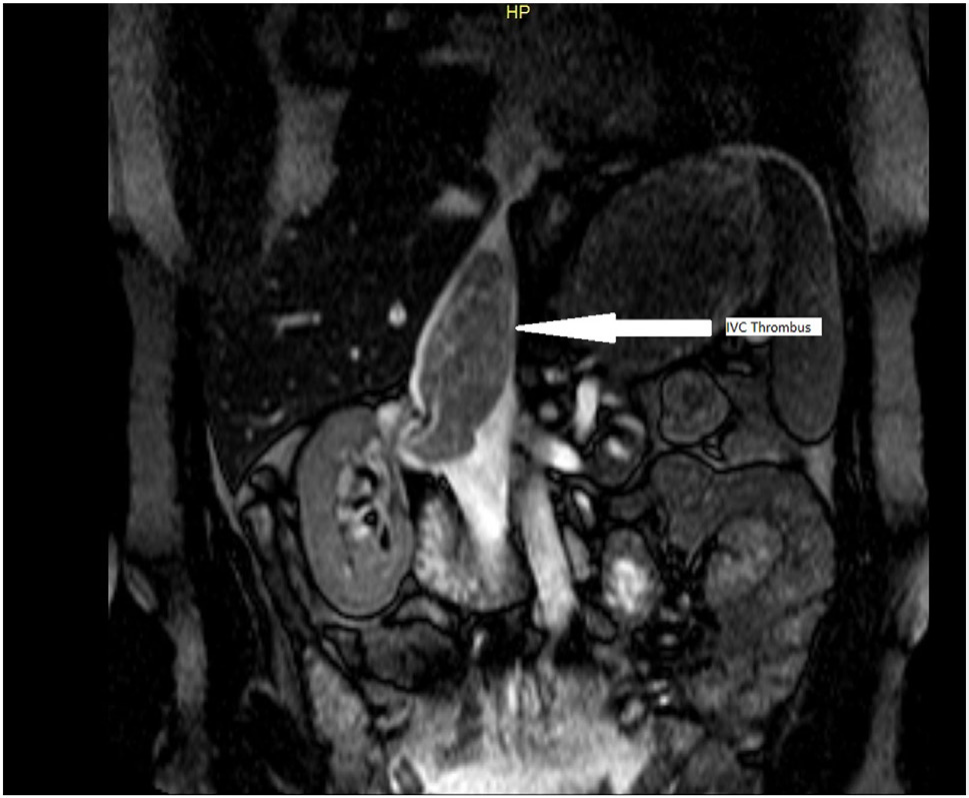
Magnetic resonance imaging image showing extension of the thrombus from the right kidney into the inferior vena cava (IVC) up to the level of the diaphragm, arrow showing IVC thrombus.
